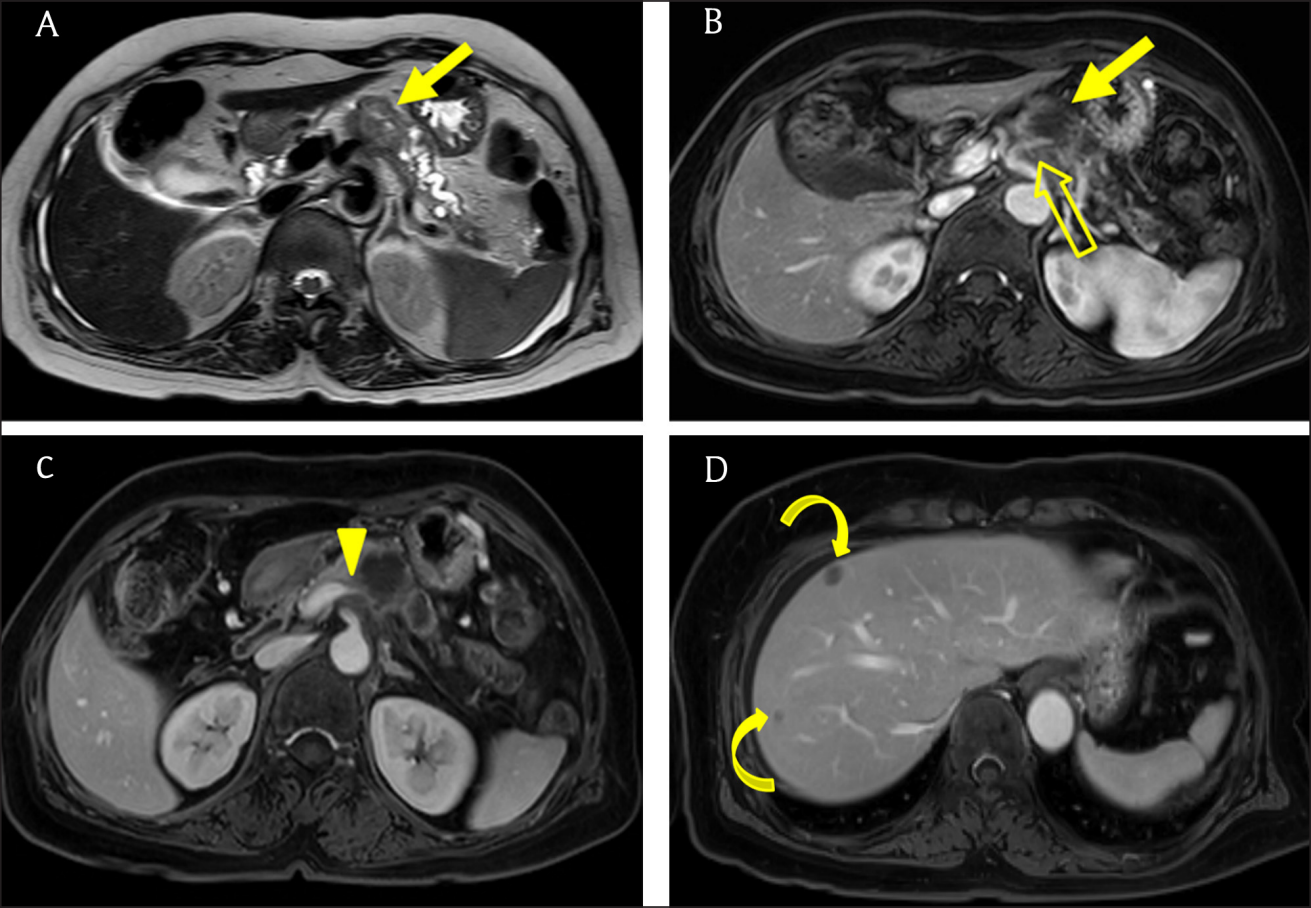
A 60-year-old woman with pancreatic ductal adenocarcinoma. (A) Axial T2-weighted image shows a hyperintense mass (arrow) in the pancreatic body with upstream dilatation of the pancreatic duct. (B, C) Axial gadoxetic acid-enhanced arterial and portal venous phase MR images show a hypovascular mass (arrow) that invades the splenic artery (open arrow) and vein (arrowhead). (D) Axial portal venous phase MR image shows hypovascular metastatic lesions (curved arrows) in the liver.
